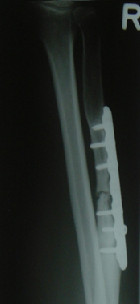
X-ray of the right forearm 19 months after ORIF of a 34 year old man showing a persistent non-union of the ulna.
